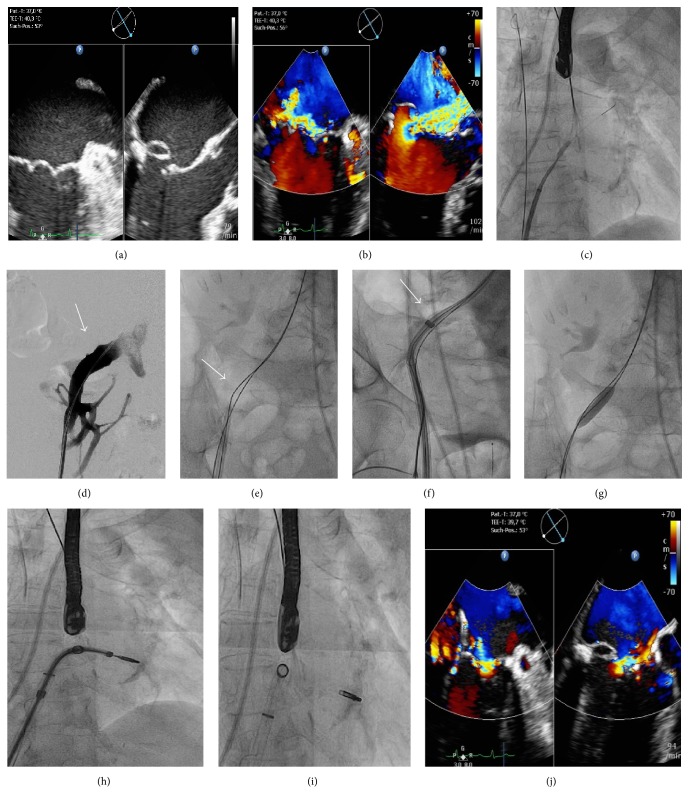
Images of transesophageal echocardiography (TEE) and fluoroscopy during percutaneous edge-to-edge mitral valve repair (PMVR)*. (a) The biplane intercommissural and left ventricular outflow tract (LVOT) view in TEE showing a* severe* prolapse and flail of the posterior mitral leaflet (PML; P2 segment). (b) The biplane intercommissural and LVOT view in TEE with color Doppler demonstrating eccentric severe mitral regurgitation (MR). (c) Fluoroscopy showing balloon dilatation of the interatrial septum over a transseptal guidewire. (d) Digital substraction angiography (DSA): a mechanical obstruction in this area with a tortuous right common iliac vein precluded advancement of the MitraClip system (the depicted arrow may indicate a venous valve). (e) Fluoroscopy showing an unsuccessful attempt to dilate the right iliac vein with a dilator. (f) Unsuccessful attempt to advance the guide through the iliac vein (the arrow indicates an obstruction at the curve of the right common iliac vein). (g) Dilatation of the right iliac vein with increasing sizes of percutaneous transluminal angioplasty (PTA) balloons (up to 10 mm). (h) Successful positioning of the MitraClip delivery system and the clip within the left atrium. (i) Clip deployment. (j) Significant reduction of MR after clip deployment as demonstrated by intraprocedural TEE.
